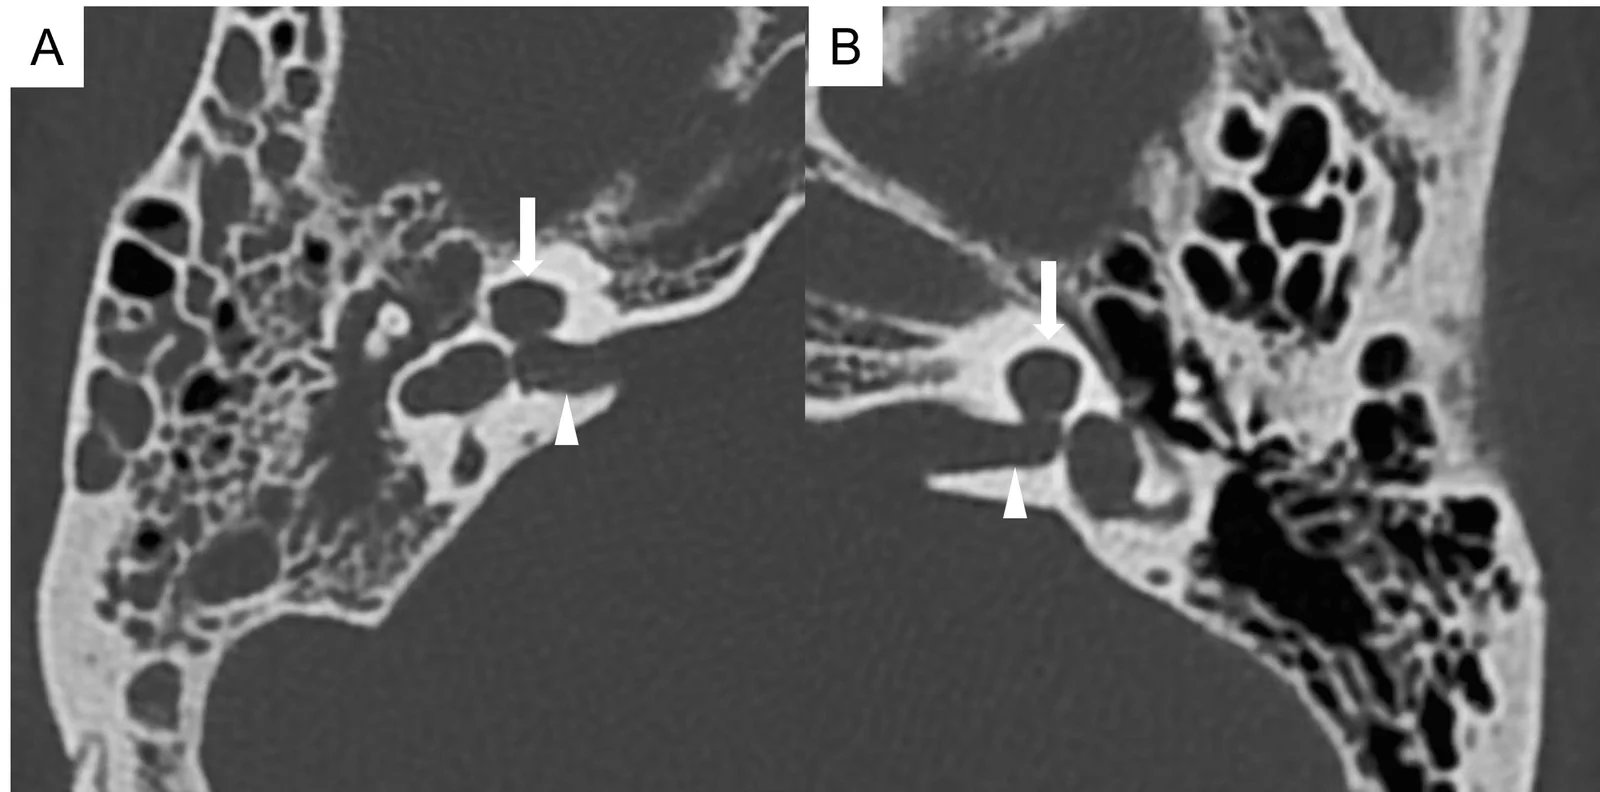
Axial CT findings of incomplete partition type I. (A) Right temporal bone: The bony partition between the internal auditory canal and the cochlea was absent, and dilation of the fundus of the internal auditory canal was observed (arrowhead). The cochlea lacked the interscalar septa and exhibited a cystic appearance (arrow). (B) Left temporal bone: Similar to the right side, dilation of the fundus of the internal auditory canal (arrowhead) and a cystic cochlea lacking the interscalar septa (arrow) were observed.
